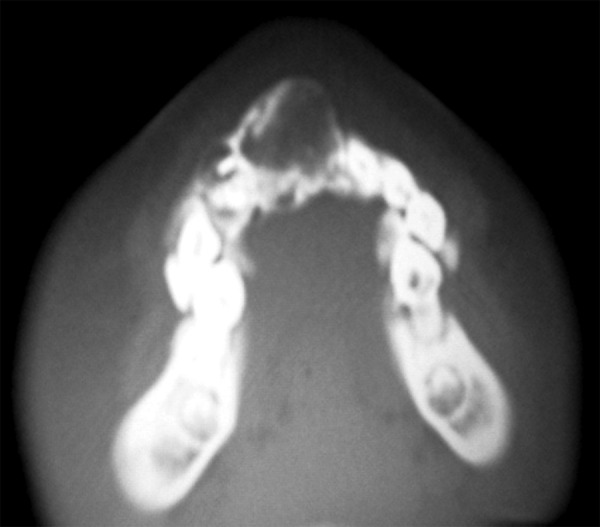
CT maxilla showing cross-sectional view of the lesion
